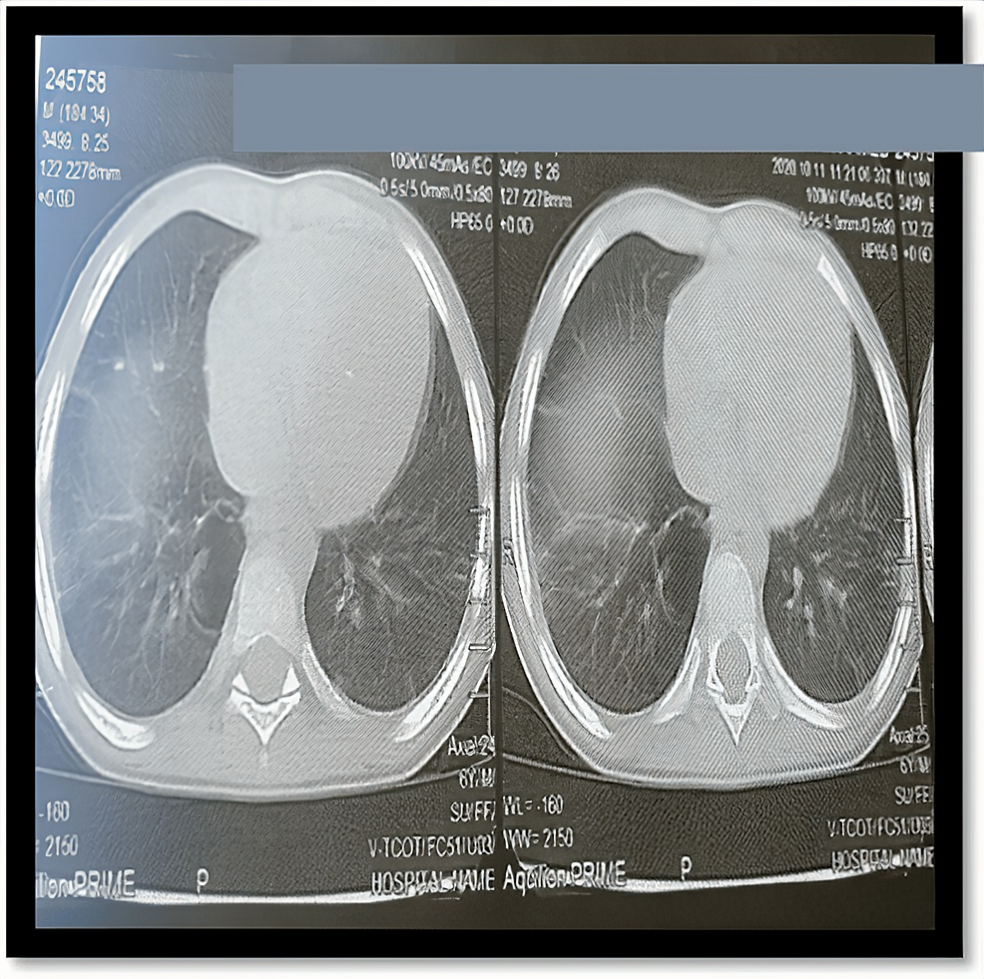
Axial computed tomography showing resolution of septic emboli seen in Figure 1.
